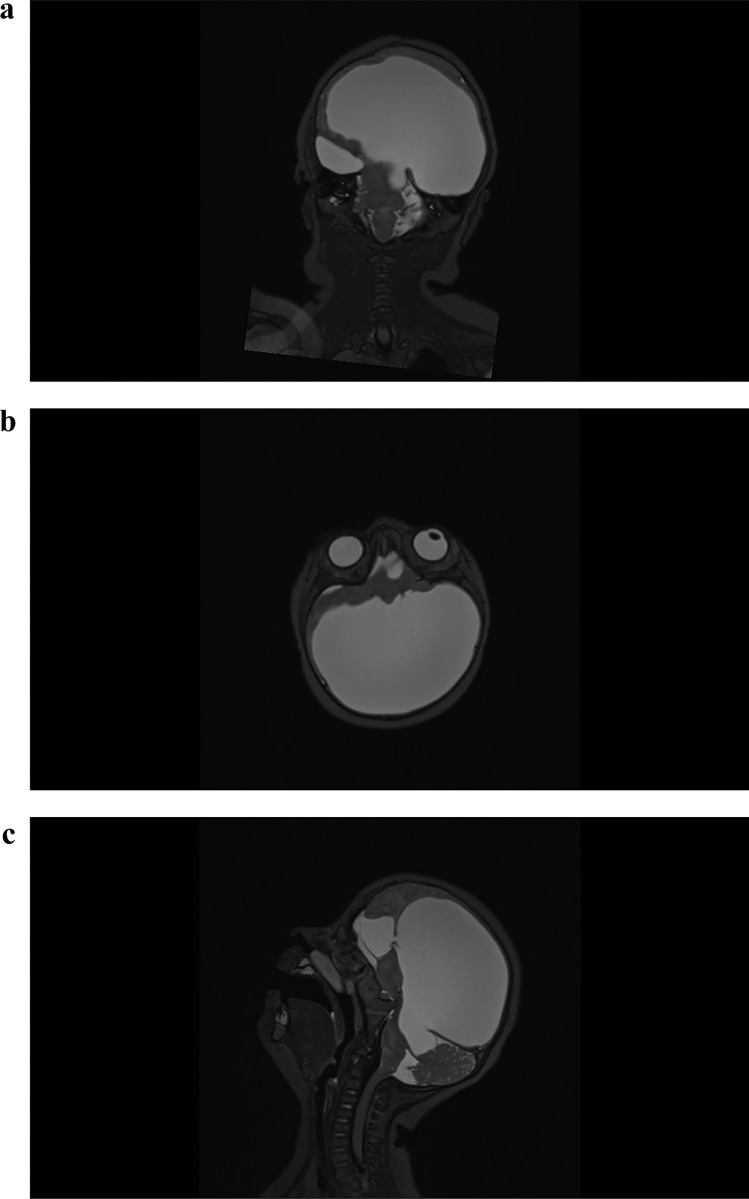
MRI Scan ( axial and coronal ) 6 months after Operation demonstrating the Hydrocephalus
